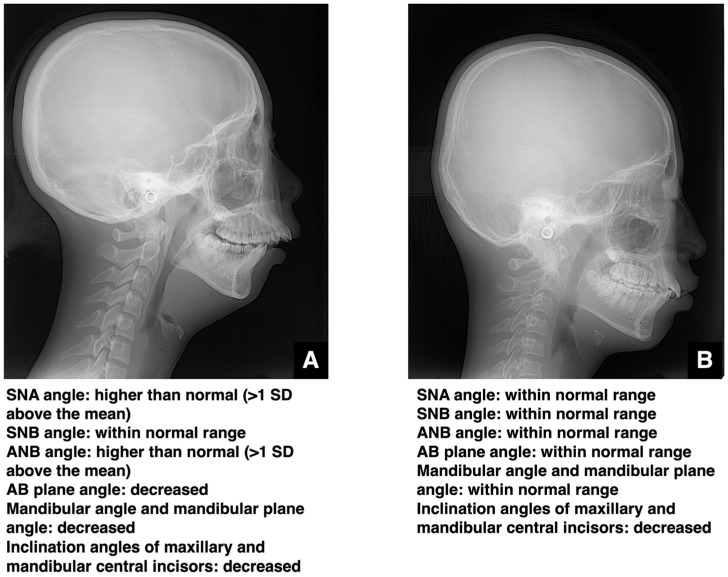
Analysis of skeletal and dentoalveolar maxillary protrusion. (A) Lateral cephalometric radiograph and summary of polygonal analysis of a patient with skeletal maxillary protrusion. (B) Lateral cephalometric radiograph and summary of polygonal analysis of a patient with dentoalveolar maxillary protrusion. Permission has been obtained to publish all figures.
